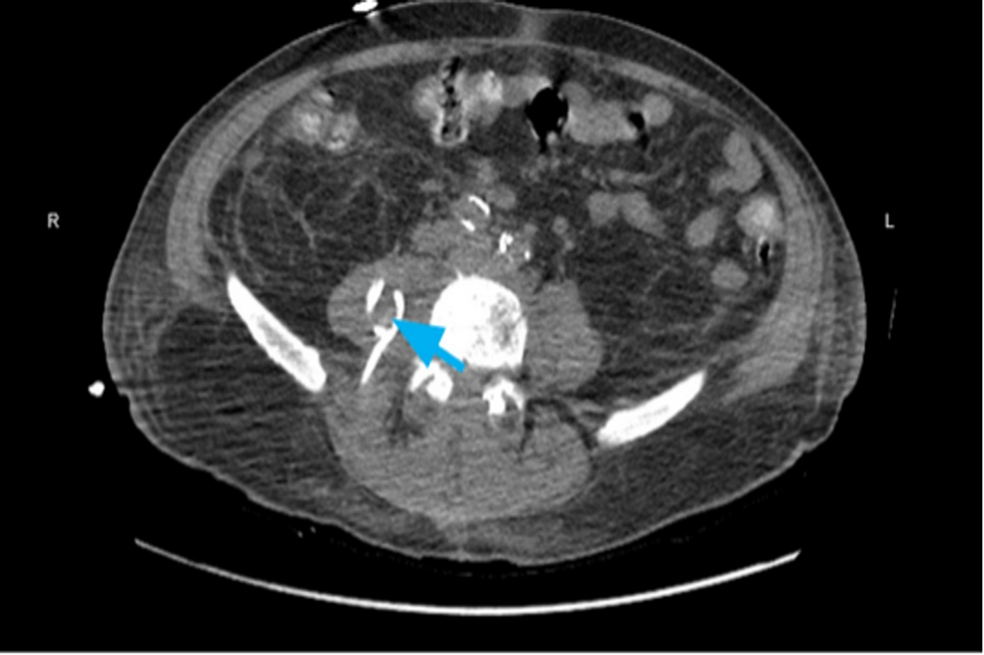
CT abdomen without contrast showing resolution of the fluid collection within the right psoas muscle after placement of a second catheter (blue arrow).
